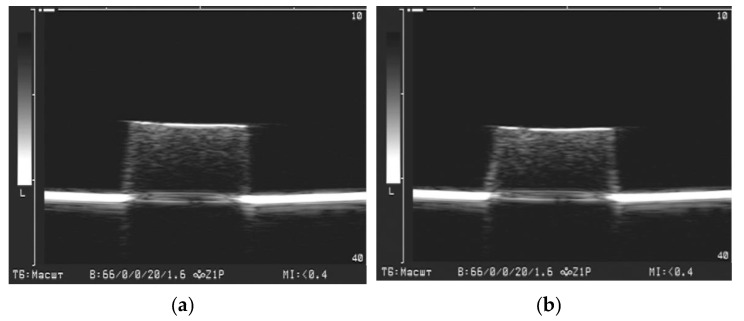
Ultrasound visualization of ferrogel deformation in applied magnetic field. FG with PAAm/Guar network and 23.3% content of iron oxide. (a) no field; (b) 400 Oe gradient field applied.
